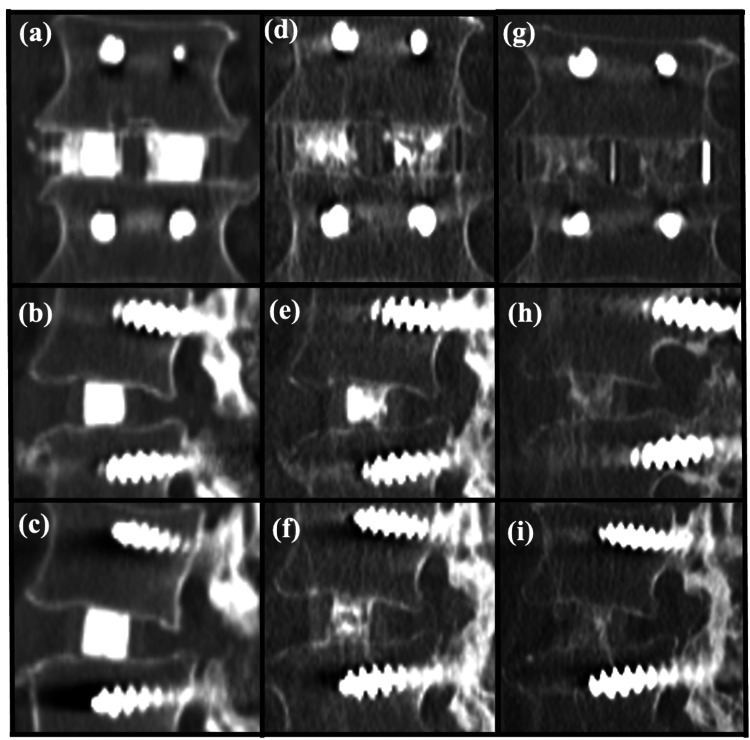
Postoperative coronal and sagittal CT scans. (a) Coronal view, (b) the right side of the sagittal view, and (c) the left side of the sagittal view: CT scans at one year postoperatively. Material resorption and newly formed bone are observed around endplates. (d) Coronal view, (e) the right side of the sagittal view, and (f) the left side of the sagittal view: CT scans at two years postoperatively. Material resorption and bone fusion were observed throughout the graft window area. (g) Coronal view, (h) the right side of the sagittal view, and (i) the left side of the sagittal view. CT, computed tomography
